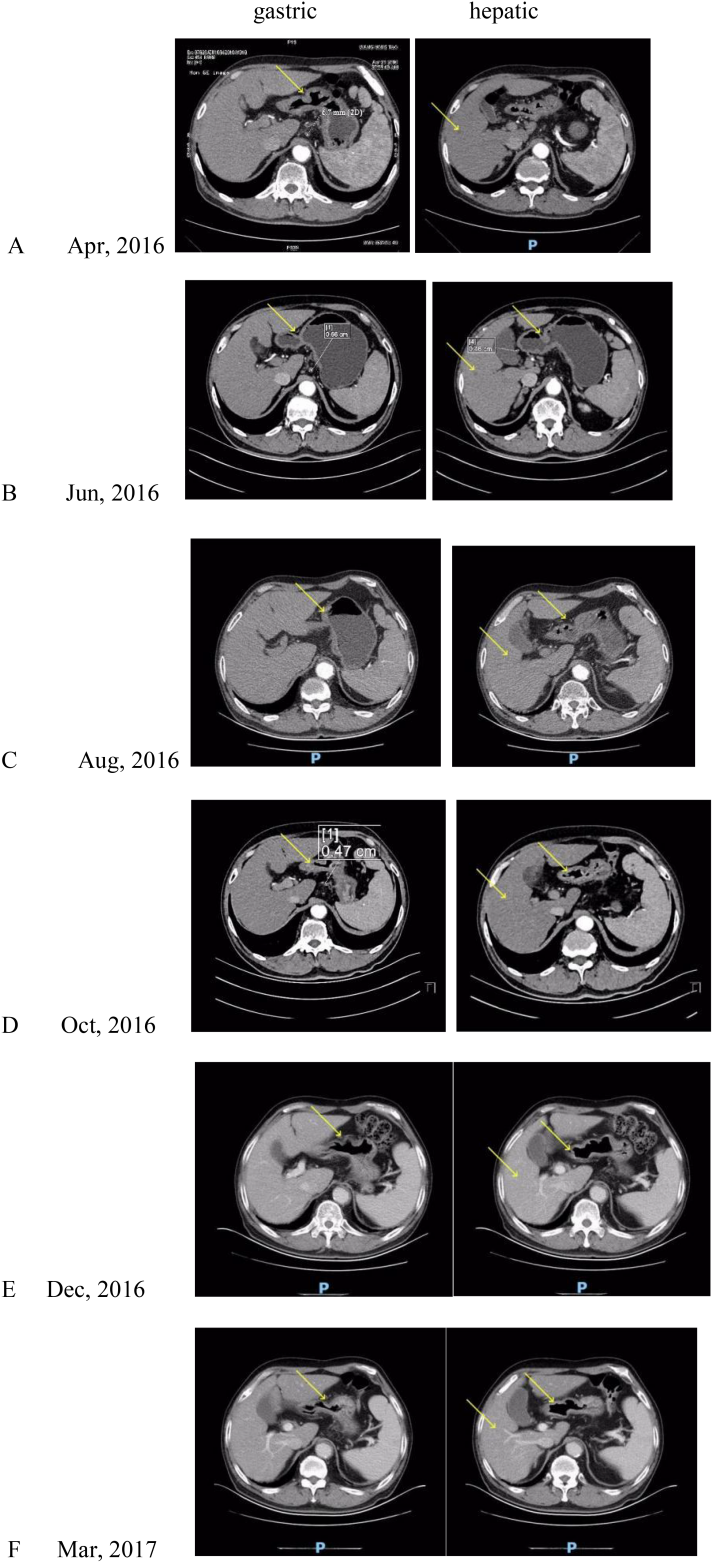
(A): CT after 1 cycle of Apatinib, (B): CT after 2 cycles of Apatinib with S-1 chemotherapy, (C): CT after 4 cycles of Apatinib with S-1 chemotherapy, (D): CT after 6 cycles of Apatinib with S-1 chemotherapy, (E): CT after 8 cycles of Apatinib with S-1 chemotherapy, (F): CT after 11 cycles of Apatinib with S-1 chemotherapy. Yellow arrows indicate the location of the lesion.
